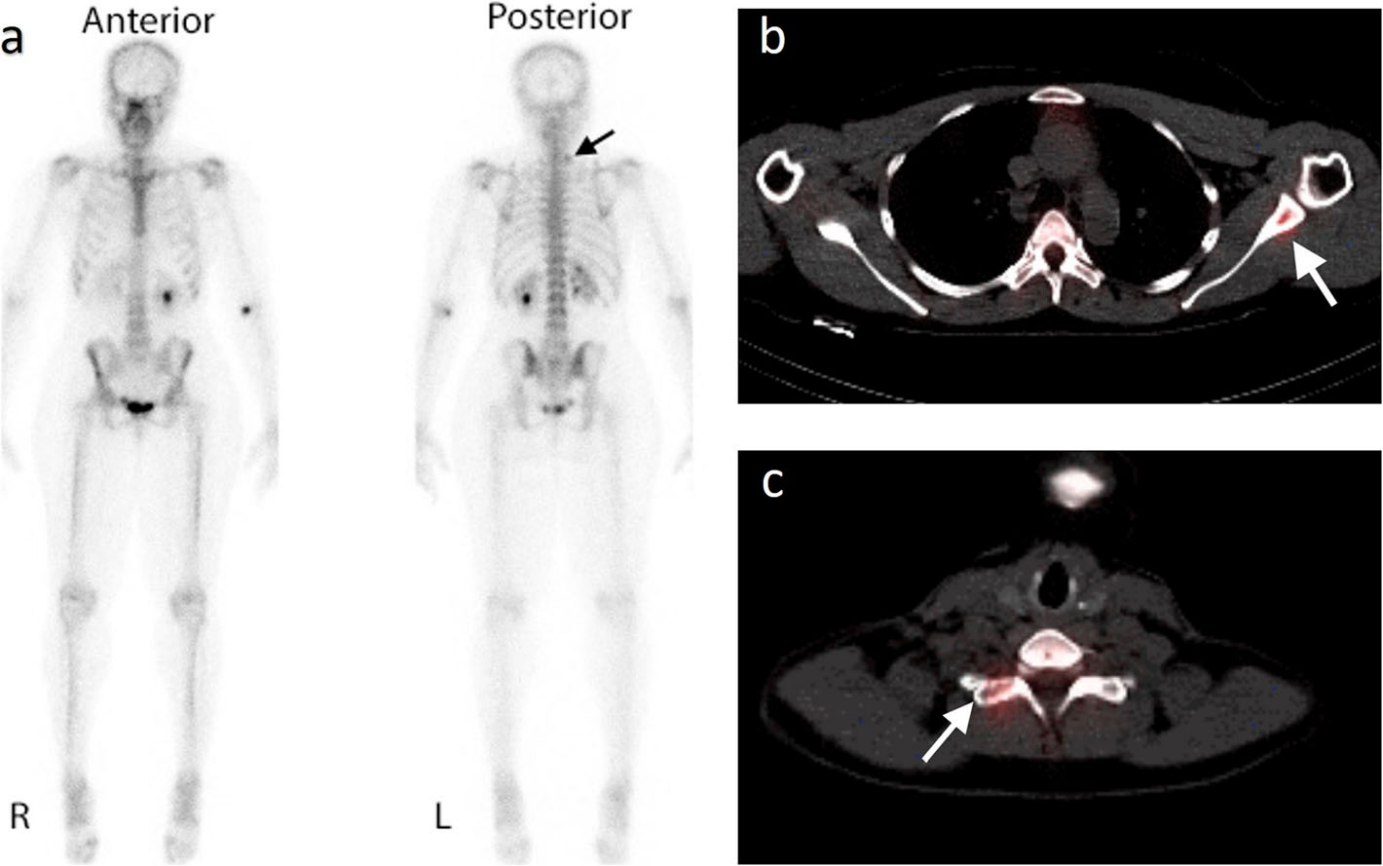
Planar bone scan versus SPECT. Images from a planar bone scan (a) and views from a single-photon emission computerised tomography (SPECT) through the thoracic (b) and cervical (c) spine in a 56-year-old woman with breast cancer. Subtle abnormal tracer accumulation is present at the root of the left neck on the planar bone scan (black arrow) with normal accumulation within the urinary tract and at the injection site (a). SPECT-CT demonstrates corresponding uptake in right transverse process of C7 (c white arrow) but additional uptake in the left scapula (b white arrow) not visible on the planar images alone
